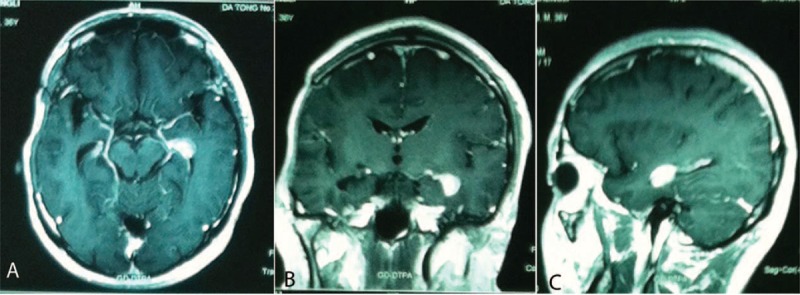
Radiological evaluation of the brain 4 months after surgery. T1-weighted axial gadolinium-enhanced magnetic resonance image demonstrates a new enhancing nearly semicircular lesion in the area of amygdala, uncus, and hippocampus.
